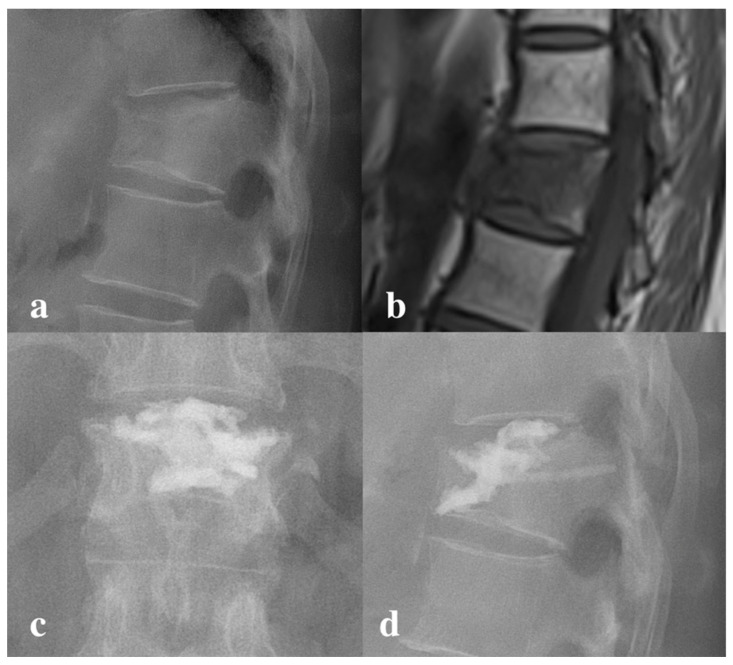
Intradiscal cement leakage. A 61 year-old female received vertebroplasty with high-viscosity cement because of the compression fracture of T12. There are no cleft signs on preoperative (a) X-ray and (b) MRI T1 weight image. Postoperative X-ray showed intradiscal cement leakage in both (c) AP view and (d) lateral view.
